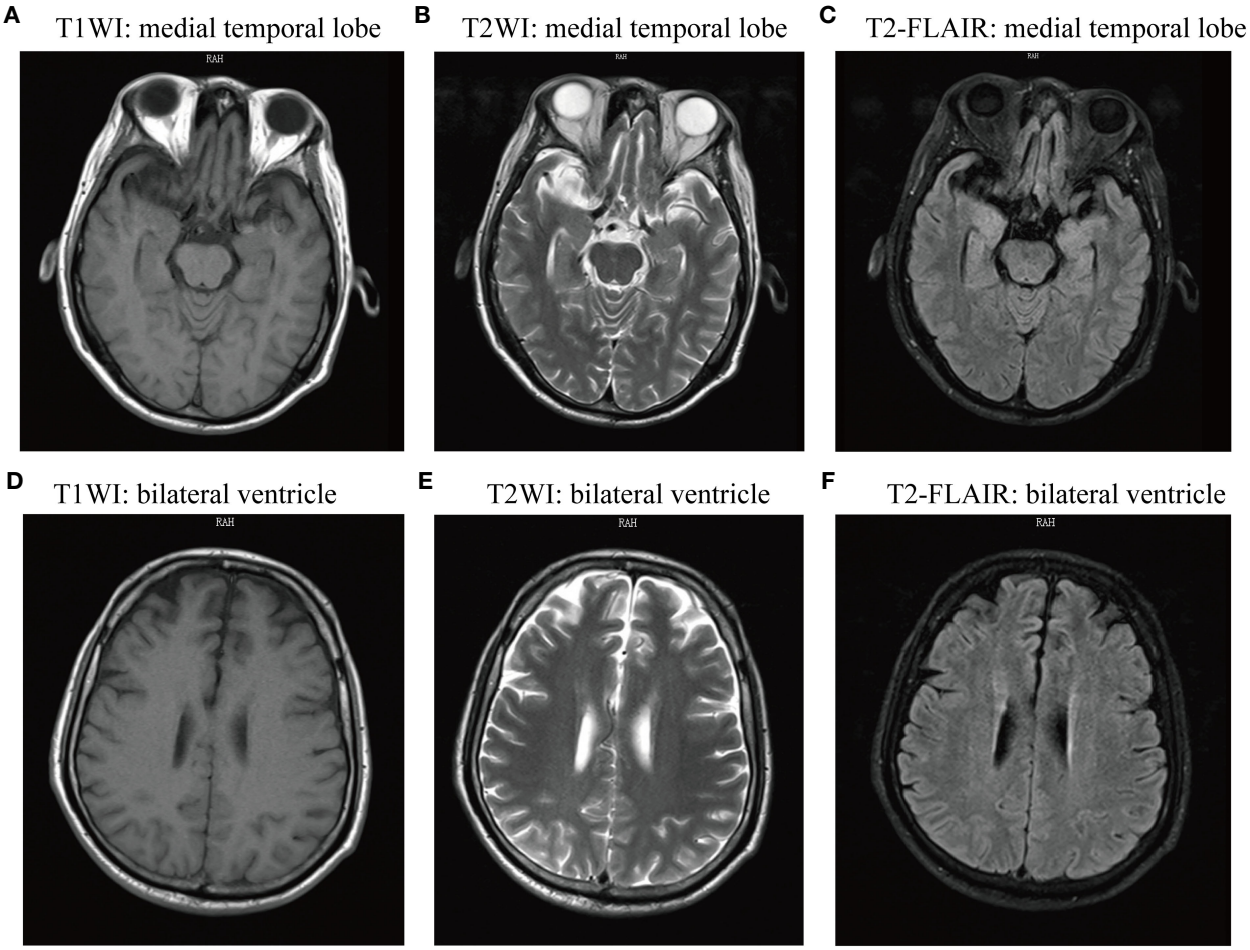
Magnetic resonance imaging (MRI) of the brain showed no abnormal signs. The two sides of the brain were symmetric, the gray matter and white matter were clearly demarcated, and there was no obvious abnormal signal shadow on T1WI, T2WI, FLAIR, and DWI axial view. (A-C) T1WI, T2WI, and T2-FLAIR axial view presenting medial temporal lobe; (D-E) T1WI, T2WI, and T2-FLAIR axial view presenting lateral ventricle. T1WI, T1-weighted imaging; T2WI, T2-weighted imaging; T2-FLAIR, T2-weighted fluid attenuated inversion recovery.
